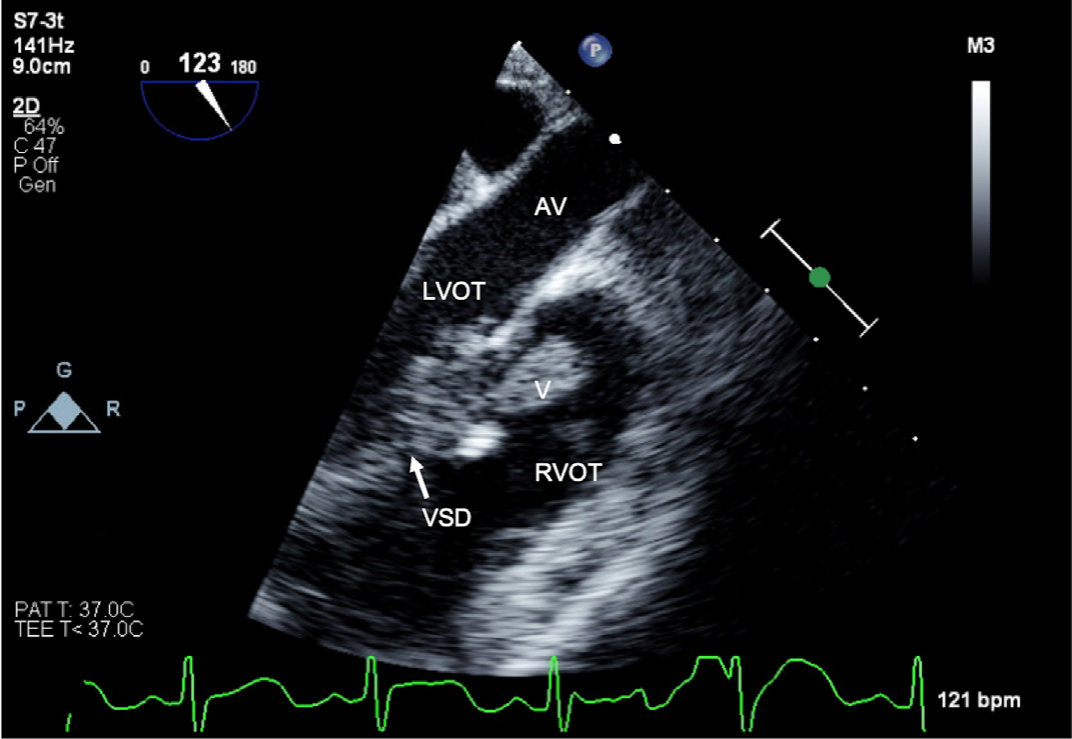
Transesophageal Echocardiogram of Several Vegetations Long-axis view demonstrating a vegetation in the right ventricular outflow tract (RVOT), adjacent to the ventricular septal defect (VSD). AV = aortic valve; LVOT = left ventricular outflow tract.
